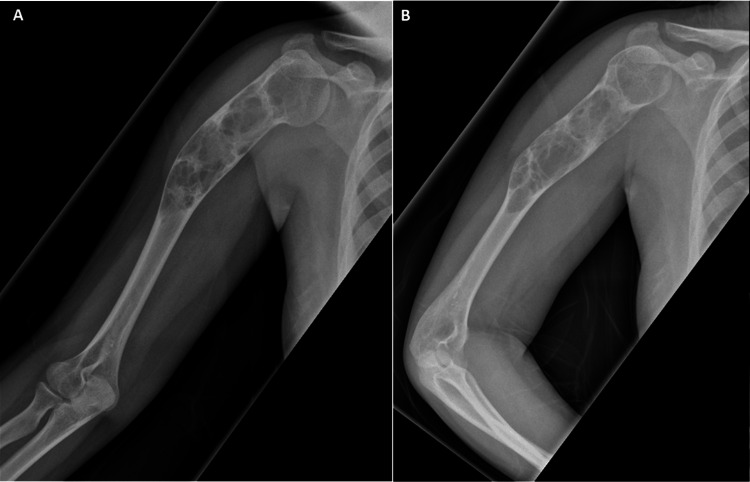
Radiograph of right humerus in anteroposterior (A) and lateral view (B) A: Anteroposterior view, B: Lateral view
